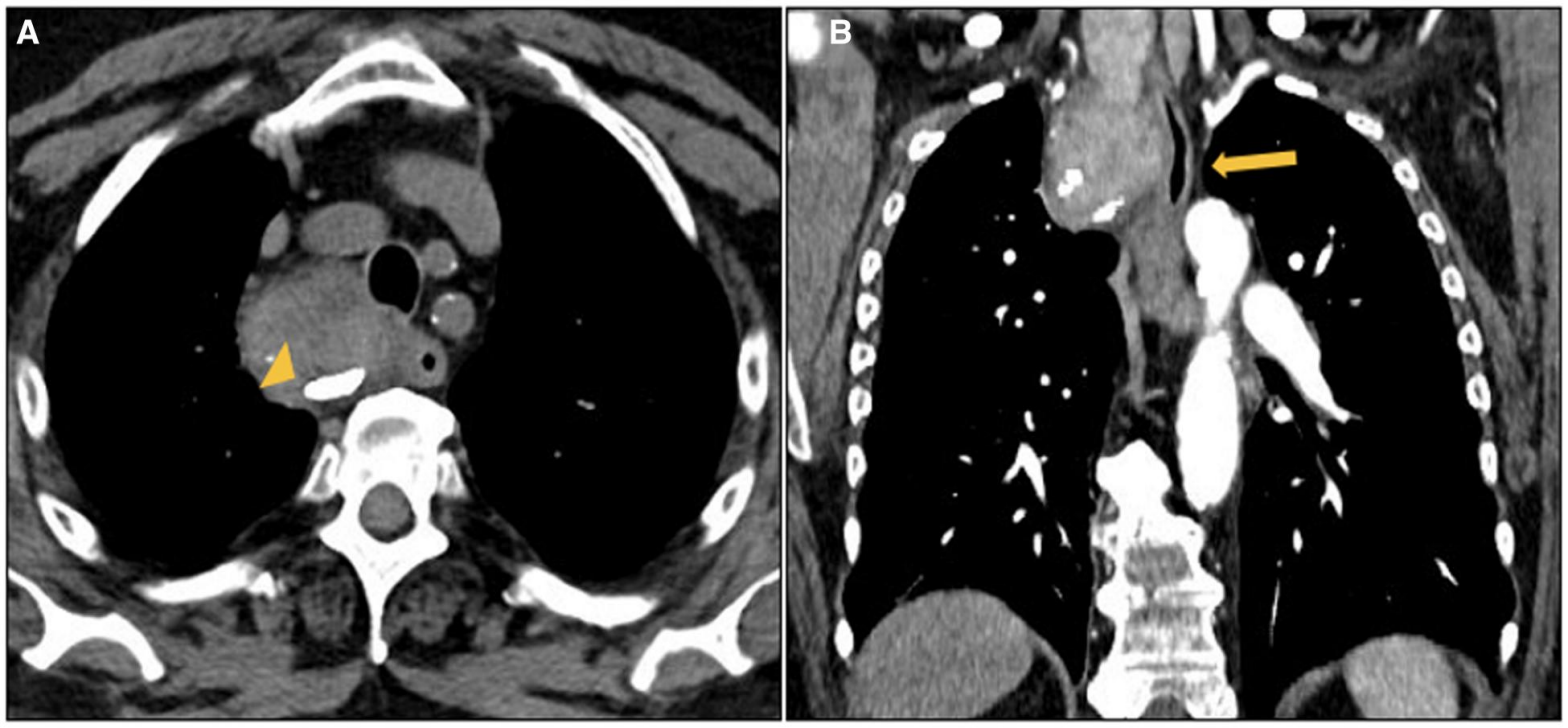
Thyroid goitre. (A) Non-contrast axial CT shows a large, lobulated mass in the visceral mediastinum with cystic changes and peripheral calcifications (arrowhead). (B) Coronal contrast-enhanced CT reconstruction shows the continuity with the right lobe of the cervical thyroid and the compression of the tracheal lumen (arrow).
